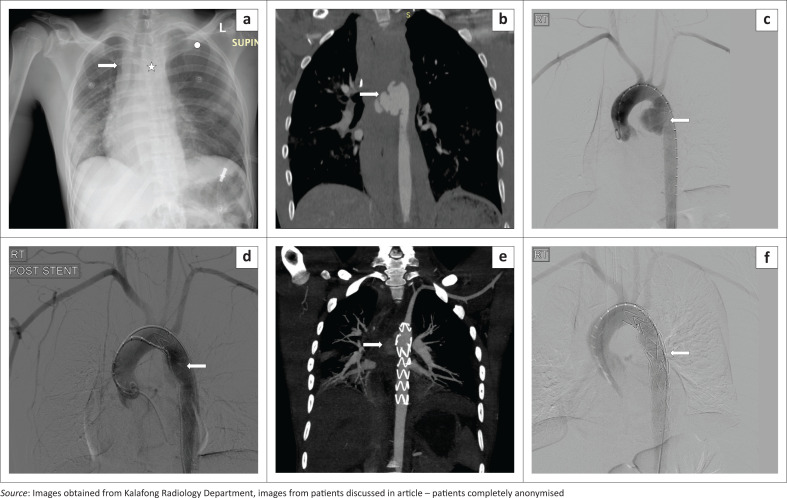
(a) Chest radiography in a 22-year-old male involved in a motor vehicle accident as a front passenger with a widened right paraspinal line (arrow), widened mediastinum (star) and depression of the left main bronchus, all signs pointing towards a vascular injury. Also note the tension pneumothorax on the left (circle) with contralateral mediastinal shift. (b) Computed tomography angiography in the same patient indicating the aortic injury and false aneurysm (arrow) at the isthmus. (c) Initial digital subtraction angiogram image pre-stenting revealing the pseudoaneurysm (arrow). (d) Digital subtraction angiogram image with the stent in place (arrow). (e) Follow-up computed tomography angiography demonstrating a leak around the stent (arrow). (f) A second endovascular stent was placed to treat the endoleak, sagittal oblique digital subtraction angiogram image with double stents (arrow).
